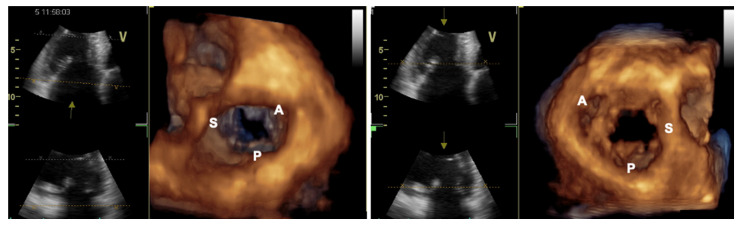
Transthoracic three-dimensional echocardiogram documenting tricuspid valve leaflets from atrial (left panel) and ventricular (right panel) perspectives. A = anterior leaflet, S = septal leaflet, P = posterior leaflet.
